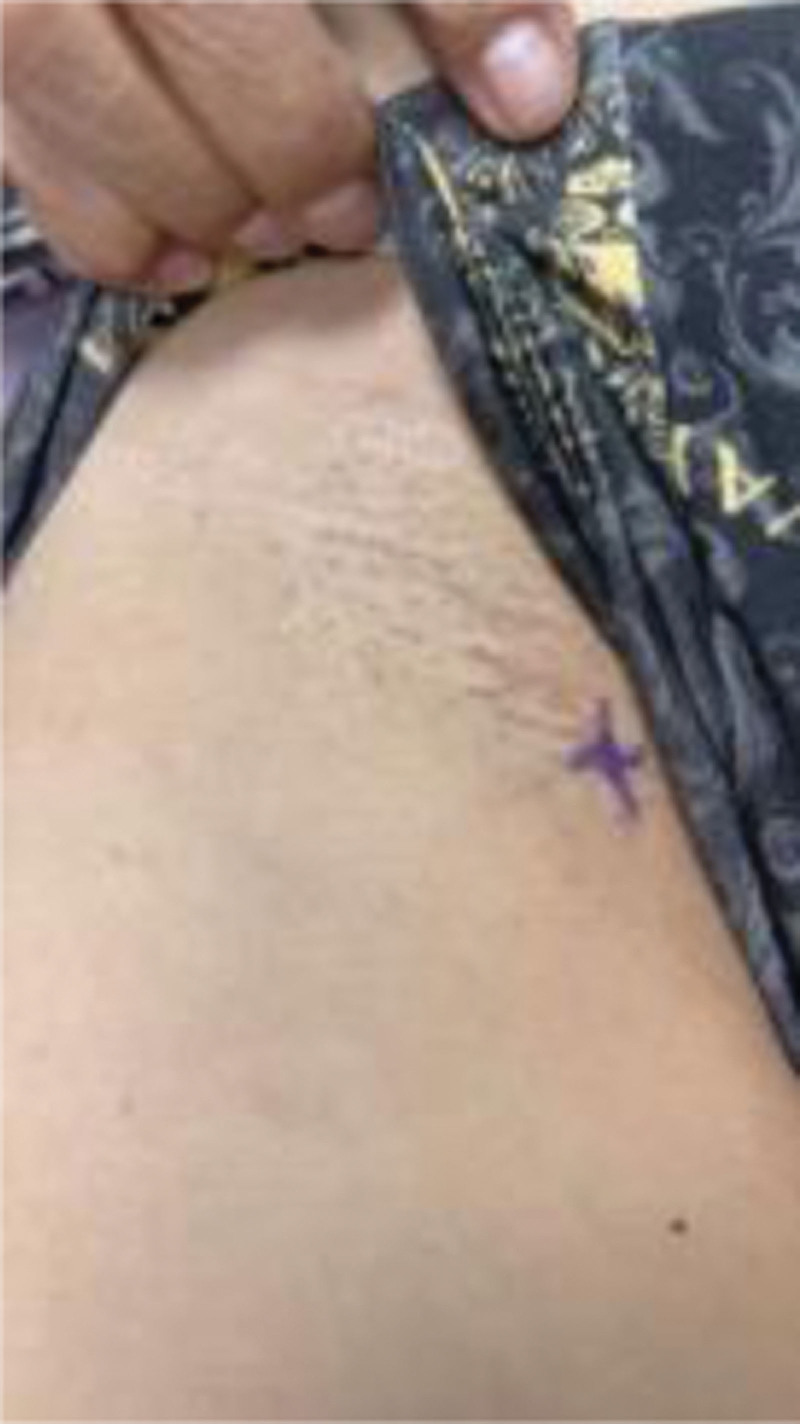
Ultrasound-guided surface marking.
